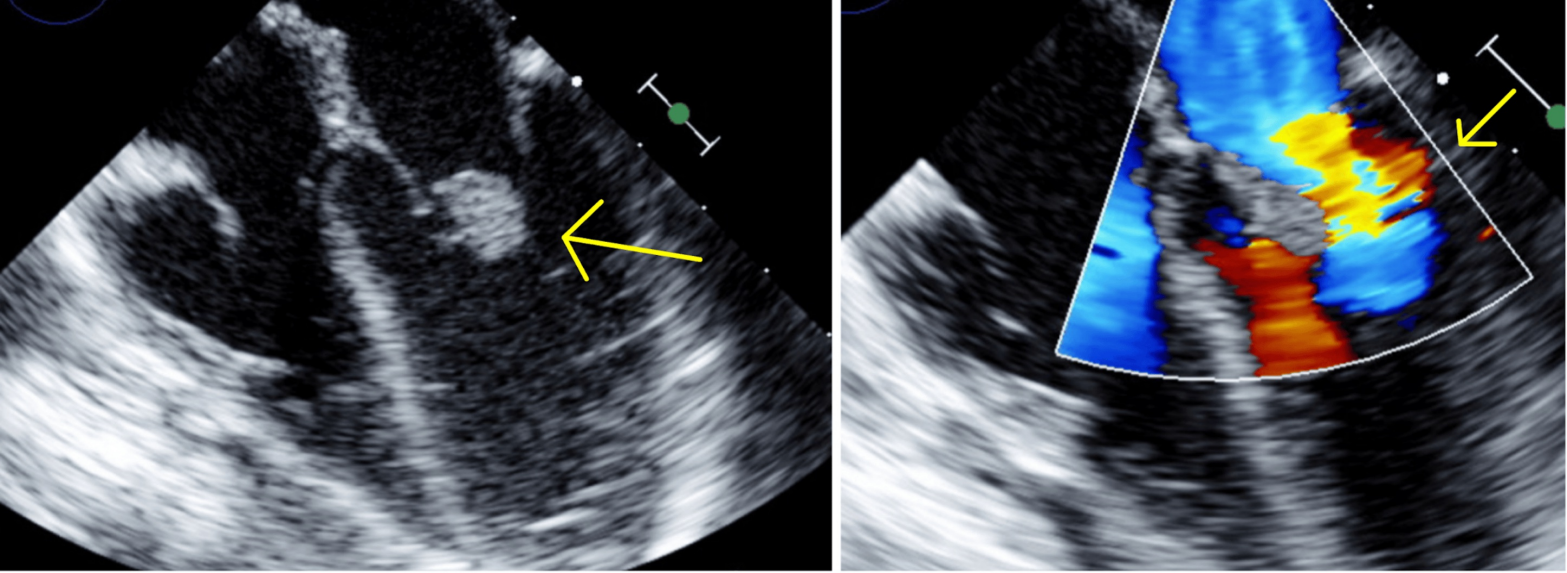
Transthoracic echocardiogram (TTE) images demonstrating a mitral valve mass (left) and regurgitation of blood flow (right).
